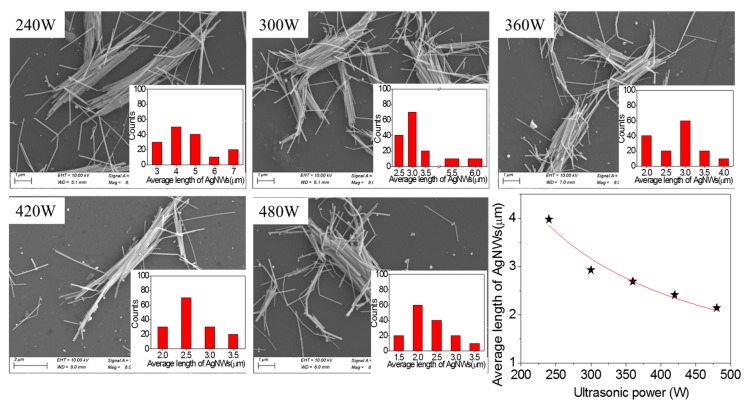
SEM images of AgNWs (1.67 mg·mL^−1^) sonicated for 1.5 h and different ultrasonic powers and the relationship of average length of AgNWs with ultrasonic power.
